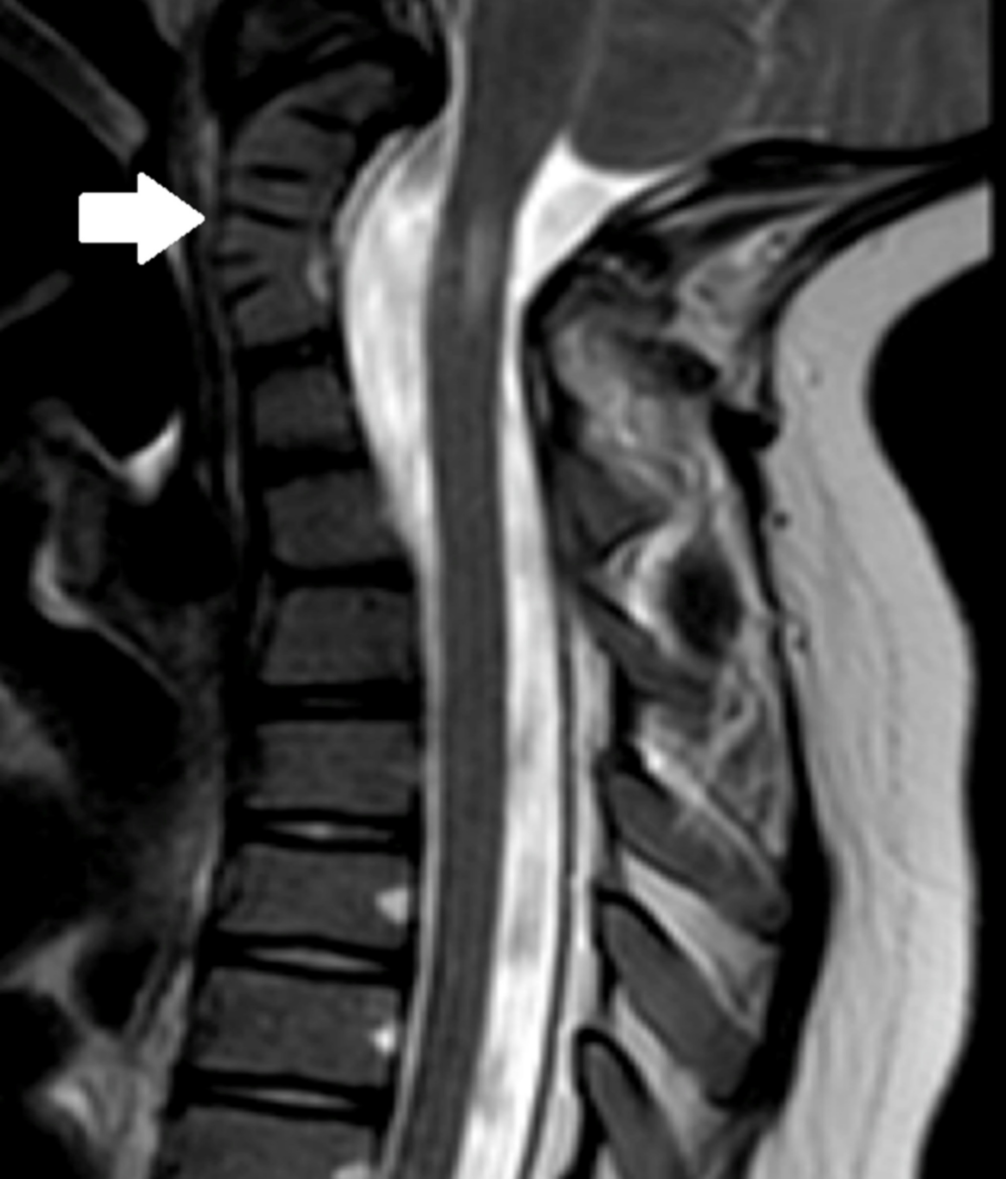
Block vertebrae Sagittal T2-weighted MRI of the cervical spine showing block vertebrae of C3/C4 (white arrow); it may or may not cause hyper- or hypomobility in translation or rotational motion adjacent to the block but can pose a significant challenge to surgery of the cervical spine [18].
